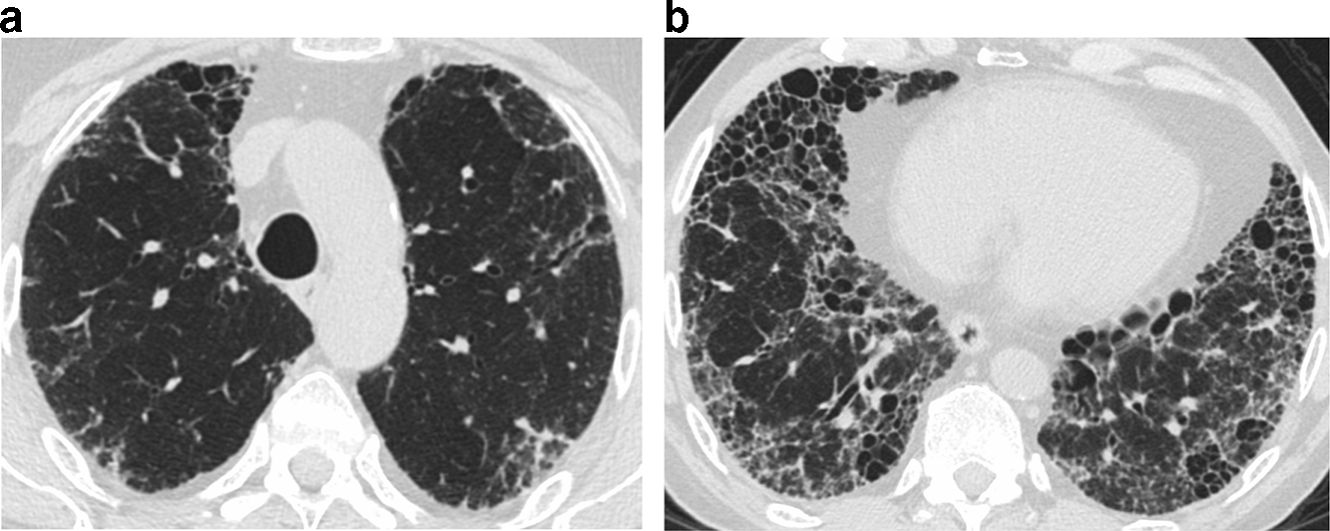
Typical UIP on HRCT. Axial images at cranial (a) and caudal (b) level show subpleural fibrosis with reticulation, traction bronchiectasis, and honeycombing. The extent of fibrosis is worse at the more caudal level, consistent with lower lung predominance of fibrosis. The basal and subpleural distribution and associated honeycombing are requisite for typical UIP. HRCT, high-resolution CT; UIP, usual interstitial pneumonia.
